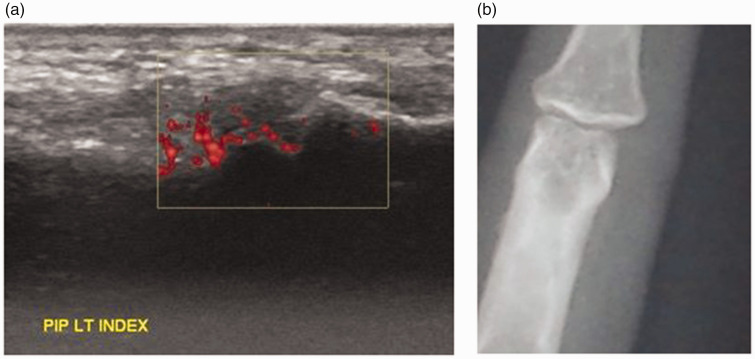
(a) Ultrasound of the 2nd PIP joint palmar longitudinal view with hypoechoic area denoting severe synovial hypertrophy grade 3 with moderate vascularization (grade 2 power Doppler signal) denoting moderate inflammation and (b) Plain radiograph of the 2nd PIP joint taken in a posteroanterior (PA) view showing juxta articular osteopenia.
